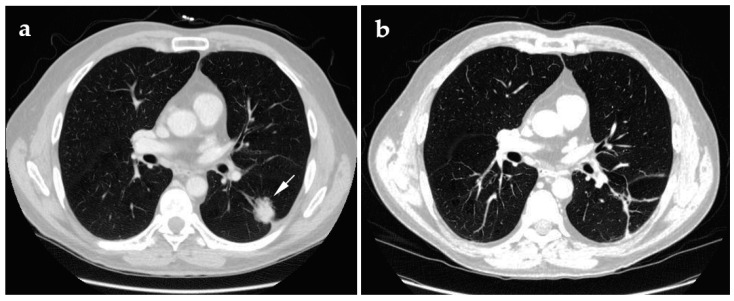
61-year-old male presenting with adenocarcinoma with invasive acinar growth. Endobronchial ultrasound showed no lymph node involvement. (a) Supine axial pre-cryoablation CT with the white arrow indicating the lesion to be ablated; (b) supine axial follow-up CT at 1-year post-cryoablation.
